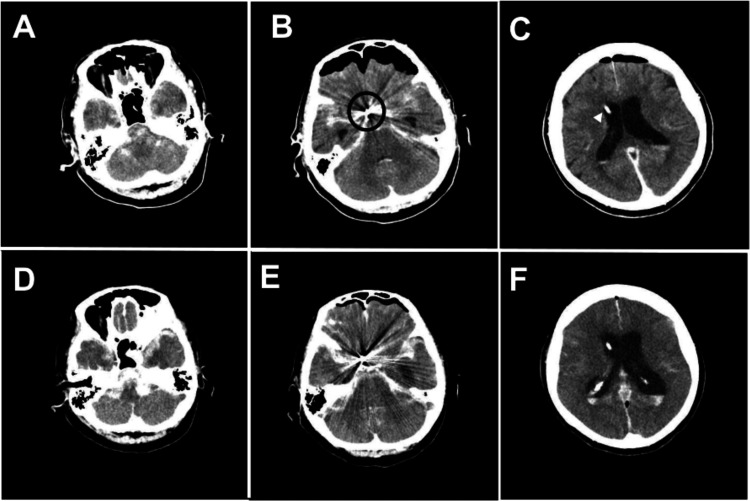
Postoperative computed tomography (CT) images. (A-C) After embolization of the right internal carotid-posterior communicating artery aneurysm (black circle), a drainage tube (white arrowhead) was placed in the anterior horn of the right lateral ventricle. Subarachnoid hemorrhage (SAH) in the posterior cranial fossa was not prominent. (D-F) CT images obtained one day after surgery showed diffuse cerebral ischemic changes. SAH in the posterior cranial fossa had increased.
